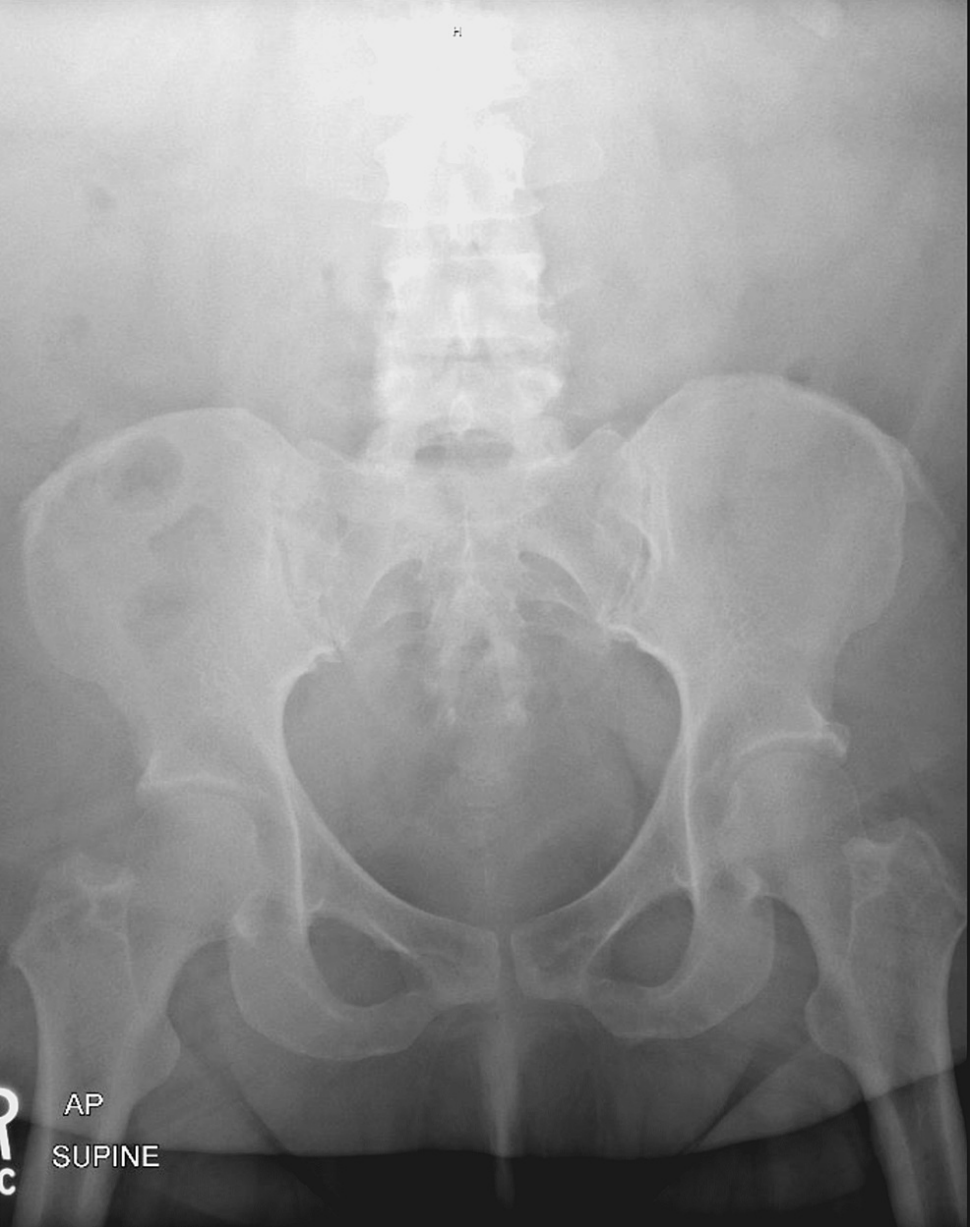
Abdominal X-Ray showed non specific gas pattern
